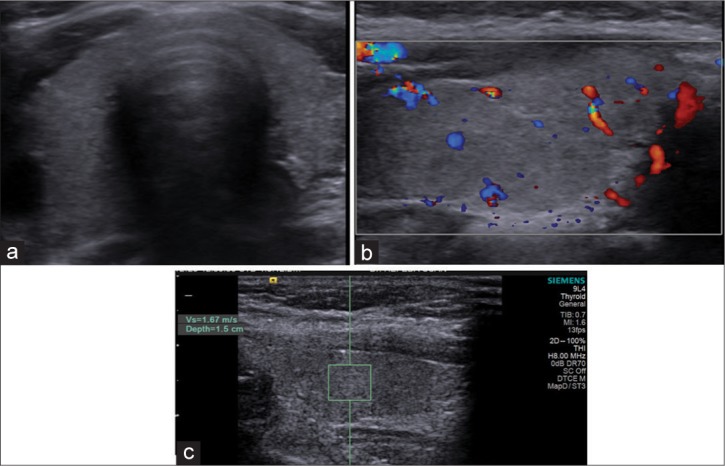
Patient with normal thyroid gland: (a) Grayscale ultrasound showing smooth homogenous isoechoic echopattern. (b) Color Doppler: Type I pattern. (c) Shear wave velocity 1.67 m/s (depth 1.5 cm).
